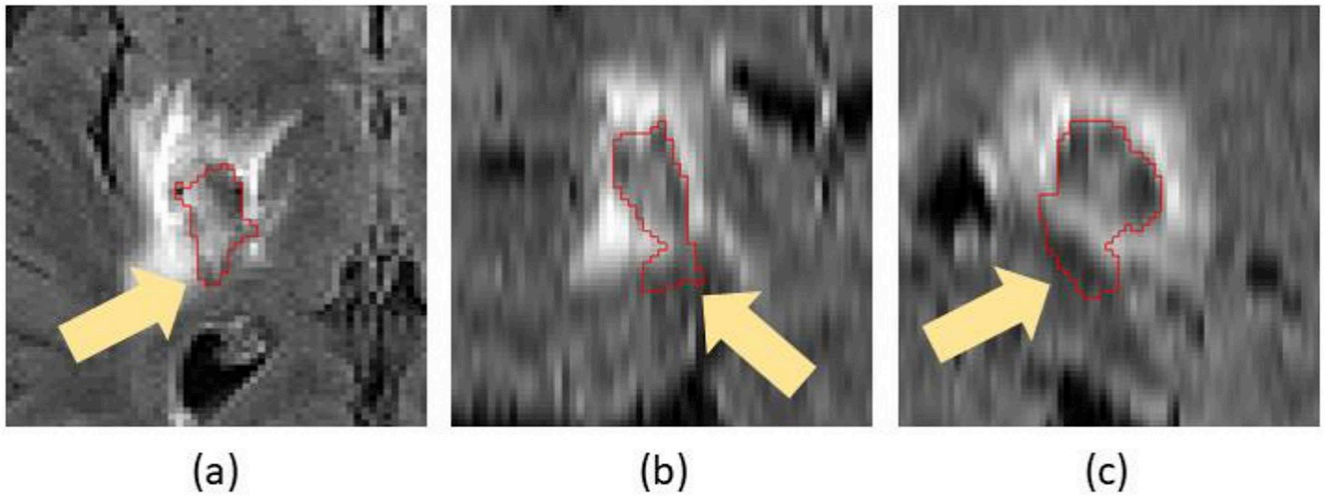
Worst case of haematoma segmentation on Dataset A. (a) FLAIR, axial. (b) FLAIR, coronal. (c) FLAIR, sagittal. Note that there is over-segmentation (yellow arrows) due to attenuated FLAIR intensities within oedema.
